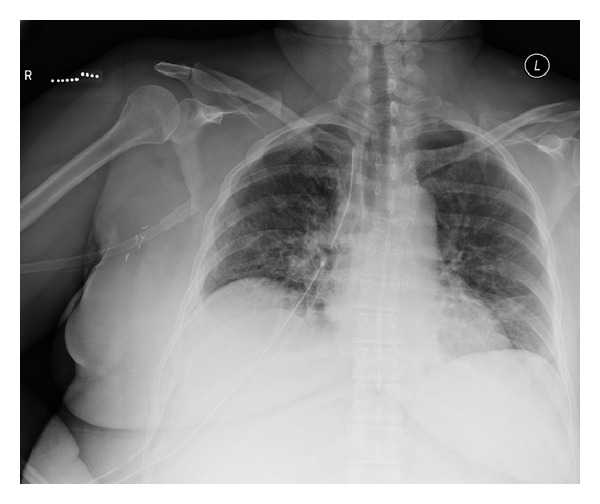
Postoperative day 7 chest X-ray. No evidence of left sided air leak or residual left main bronchus rupture.
